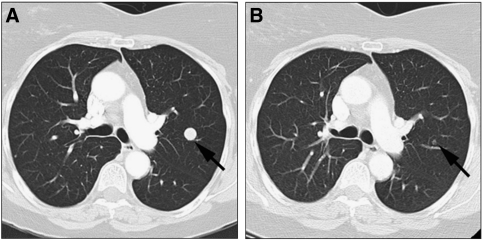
CT chest images of pulmonary metastasis arising from anal melanoma at baseline (A) and then at 3 months (B) showing reduction in the size of the lesion on imatinib (Case 1).
